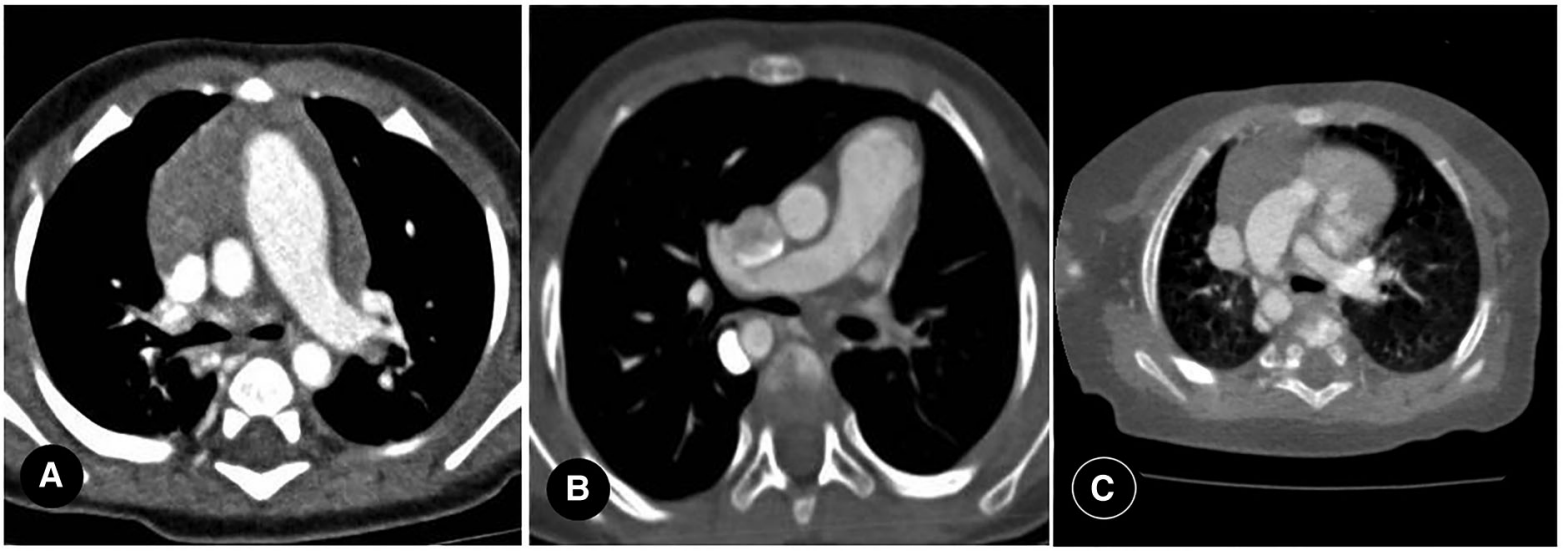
(A) right proximal interruption of the pulmonary artery (PIPA) in a 4-month-old male patient. A transverse view of the chest computed tomography angiography (CCTA) shows no indication of the mediastinal segment of the right pulmonary artery, no pulmonary artery confluence, and a right-sided hilar artery. The right internal pulmonary artery branches were open. Left-sided aortic arch. (B) left-sided PIPA in a 19-month-old female patient. A transverse view of the CCTA shows no indication of the mediastinal segment of the left pulmonary artery, no pulmonary artery confluence, and a left-sided hilar artery. The left internal pulmonary artery branches are open. Right-sided aortic arch. (C) right PIPA with a right-sided aortic arch in a 6-month-old male patient. A transverse view of the CCTA shows the absence of the right pulmonary artery with a right-sided aortic arch.
